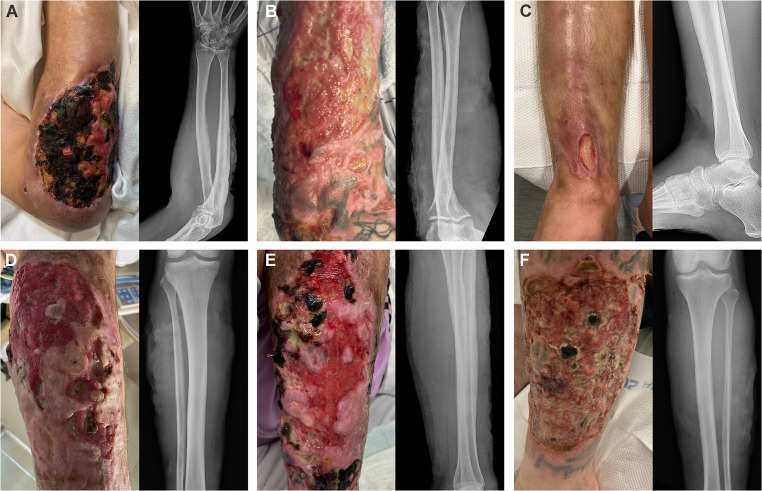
Representative clinical photographs and corresponding radiographs from six patients with xylazine-associated extremity wounds demonstrate marked irregular soft-tissue defects with ulcerated skin, undulating contours, and severe soft-tissue distortion, often disproportionate to the limited osseous findings on radiographs. (A) 56-year-old woman with an extensive forearm ulcer and overlying soft-tissue distortion; radiographs demonstrate cortical thickening of the ulna and nonspecific radial cortical thickening. (B) 39-year-old man with multiple ulcerated forearm wounds and marked skin irregularity; radiographs demonstrate mild periosteal thickening of the ulna and distal radius. (C) 43-year-old man with focal ulceration and surrounding subcutaneous edema of the lower leg; radiographs demonstrate a skin defect without cortical destruction or periosteal reaction. (D) 32-year-old woman with diffuse circumferential ulceration of the lower leg; radiographs demonstrate skin defects and limited periosteal reaction of the fibula. (E) 49-year-old woman with extensive soft-tissue swelling and irregular ulceration of the lower leg; radiographs demonstrate mild distal fibular cortical irregularity. (F) 46-year-old man with large lateral and posterior calf ulcers and severe soft-tissue distortion; radiographs demonstrate bilateral fibular periostitis without extensive cortical destruction
